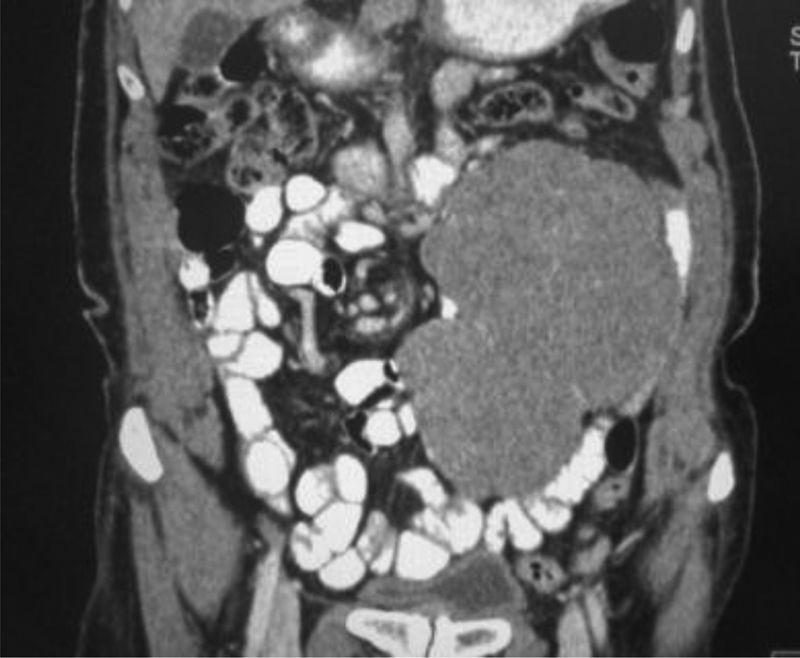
Contrast enhanced CT scan of abdomen showing the lump in the mesentery. CT, computed tomography.
